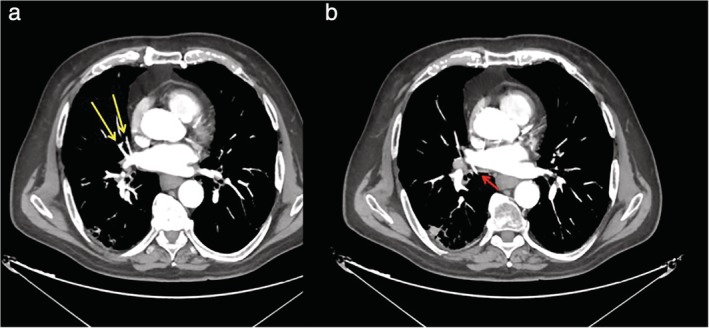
Contrast enhanced chest CT and MIP images in the assessment of venous pulmonary variants. (a) Middle lobe veins joining the superior segment right lower lobe vein (yellow arrows). (b) Independent connection of an additional superior segment right lower lobe vein direct to the left atrium (red arrow).
